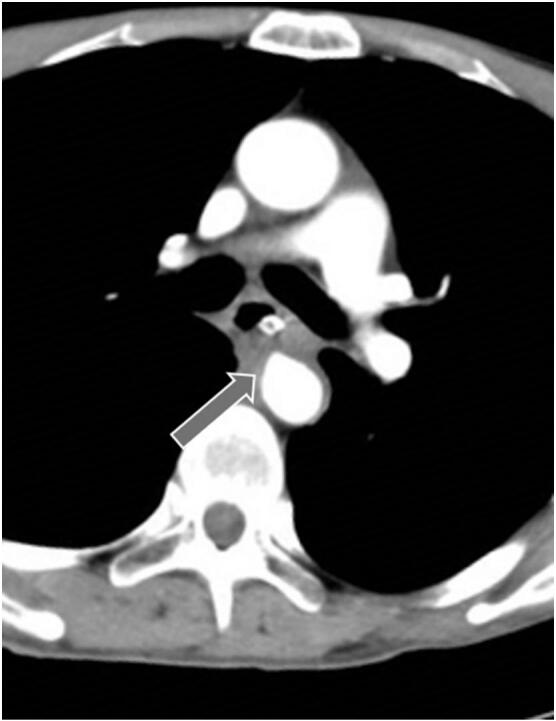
Case 1: a 51-year-old man with oesophageal fistula that developed after chemoradiotherapy for oesophageal cancer. Computed tomography performed on arrival reveals an aneurysm in the descending aorta (arrow) adjacent to the thoracic oesophagus.
